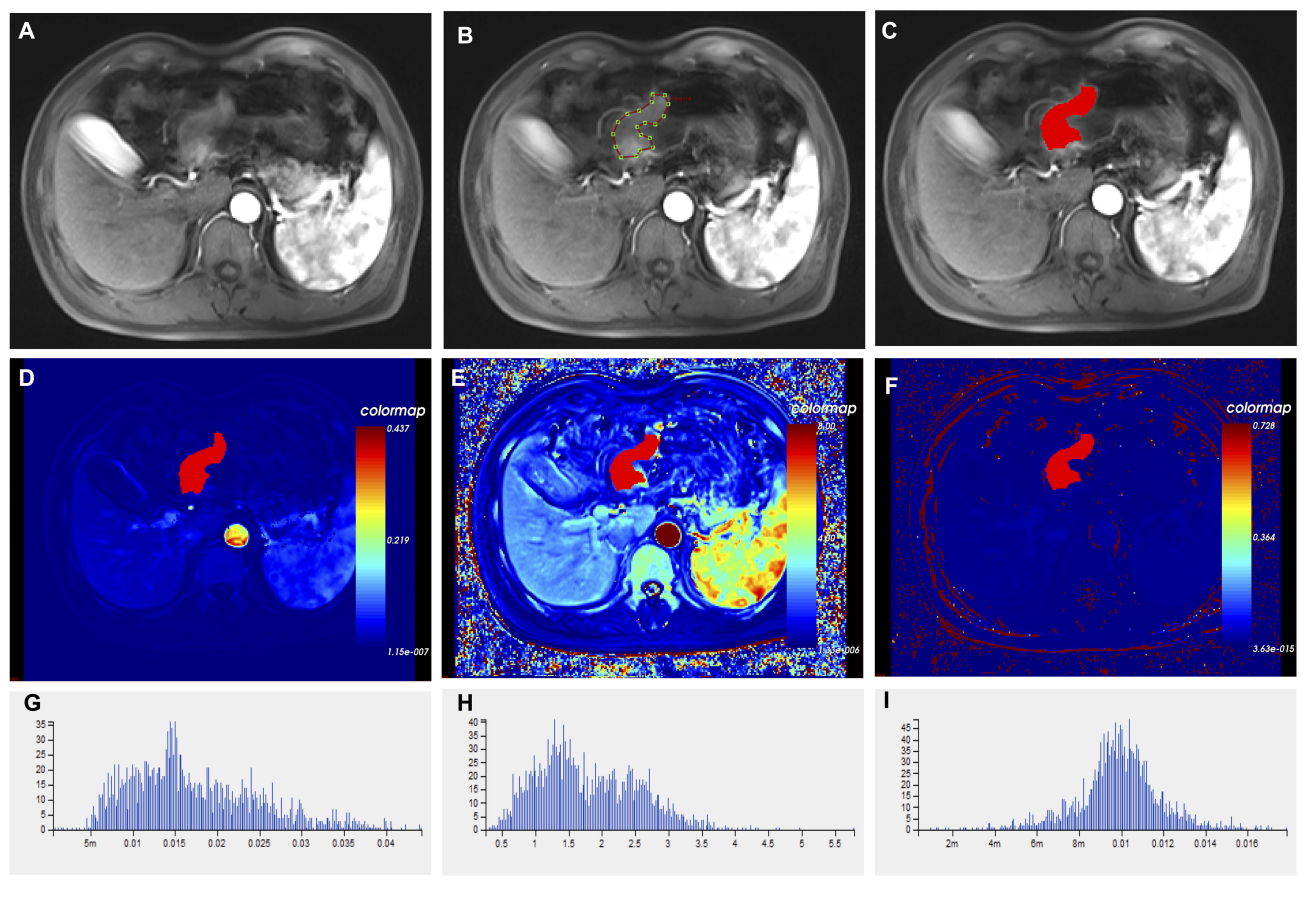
Histograms of different imaging modalities and quantitative perfusion parameters in patients with advanced gastric cancer. (A) Axial T1-weighted images showed a mass with an irregular and thickened gastric wall. (B) ROIs were placed manually in axial T1-weighted images. (C) Outlining the target area for eventual fusion into a three-dimensional structure. (D) Volume transfer constant (K^trans^) plot of the ROI. (E) The plot of the reverse reflux rate constant (K_ep_) for the ROI. (F) The plot of extracellular extravascular volume fraction (V_e_) of ROI. (G) Histogram of K^trans^ values. (H) Histogram of K_ep_ values. (I) Histogram of V_e_ values. ROI, Region of interest.
